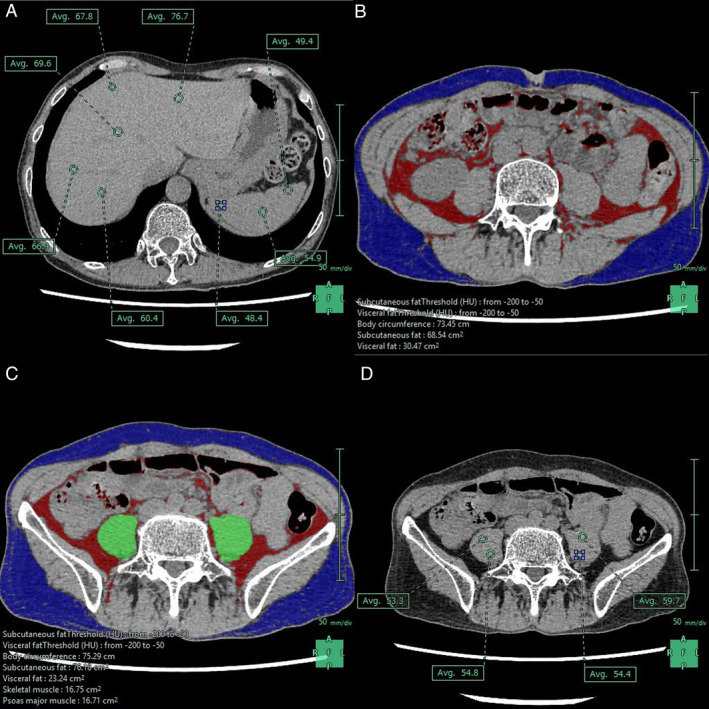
Evaluation of CT findings. (A) CT attenuation was measured at five locations in the liver and three locations in the spleen and the average value was calculated. (B) The areas of subcutaneous and visceral fat at the umbilical level were automatically calculated. (C) The psoas muscle area at the L3 level, which was added to the left and right psoas muscle areas, was also automatically calculated. (D) CT attenuation was measured at three locations on each side of the psoas muscle at the L3 level, and the average value was calculated.
